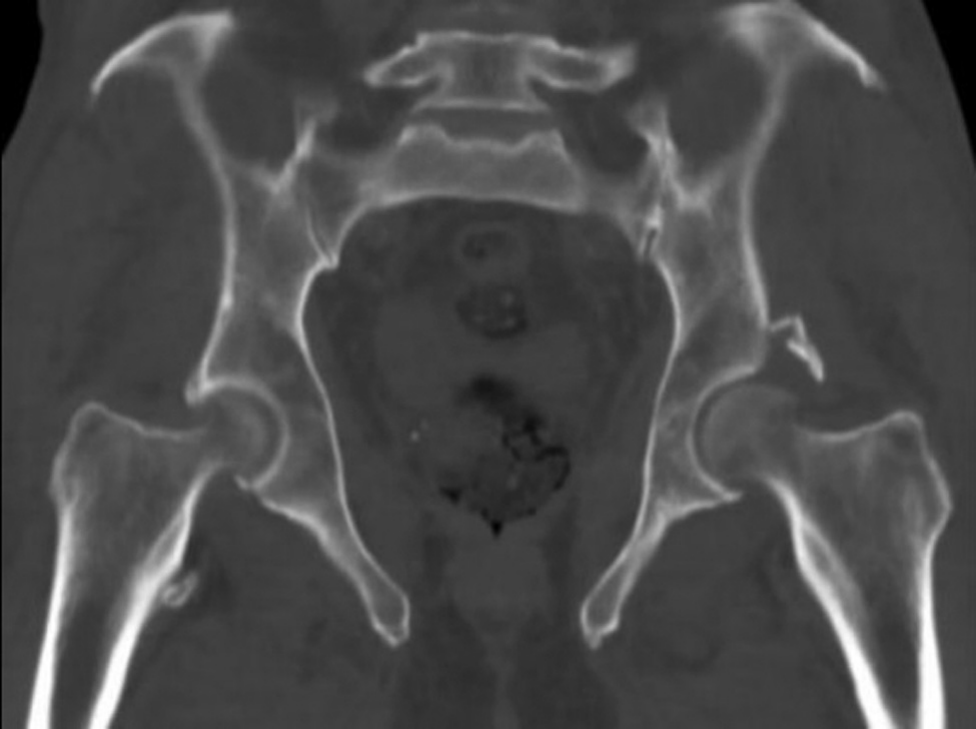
Computed tomography of the pelvis for surgical planning of fracture of the posterior wall of the left acetabulum.
